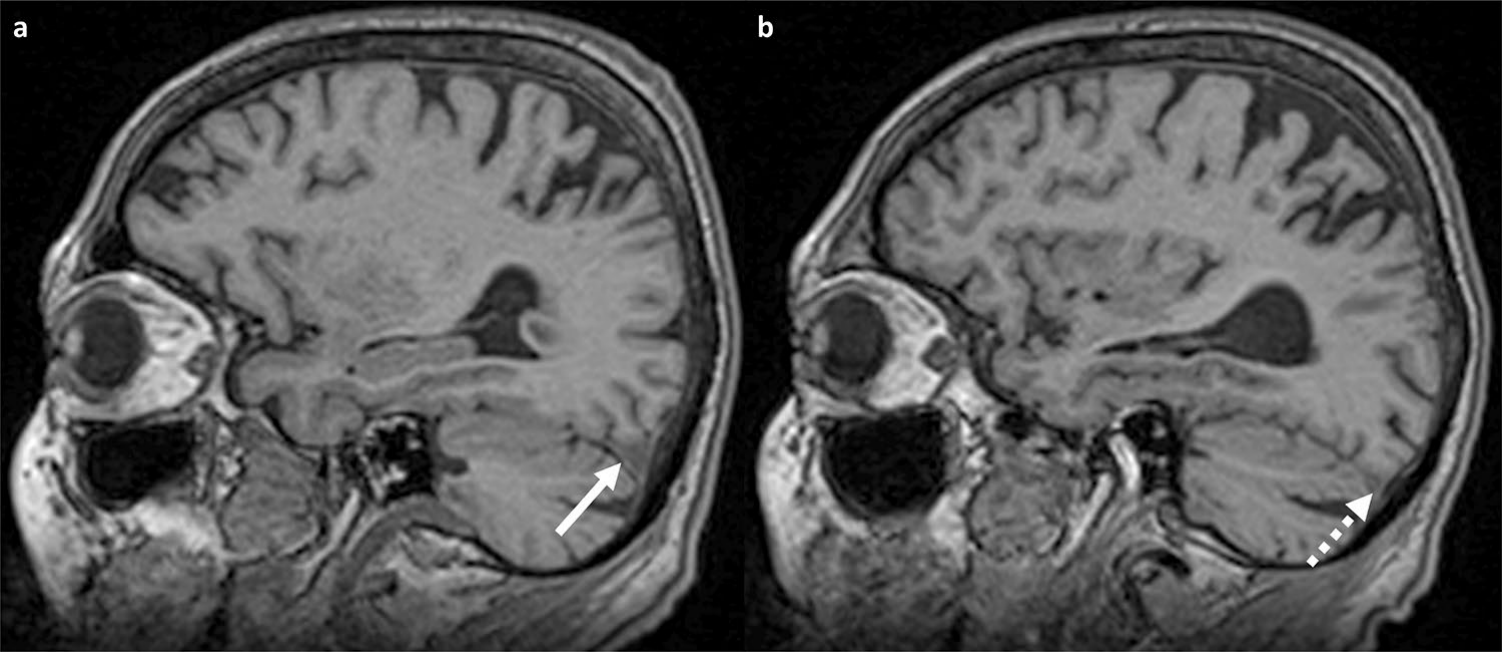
Variations of the transverse sinus on parasagittal reconstructed T1-weighted images; (a) 78-year-old female patient with normal appearing transverse sinus (white arrow). (b) 80-year-old female patient with non-detectable transverse sinus (dotted white arrow) at the identical slice position rated as occluded/stenotic transverse sinus.
